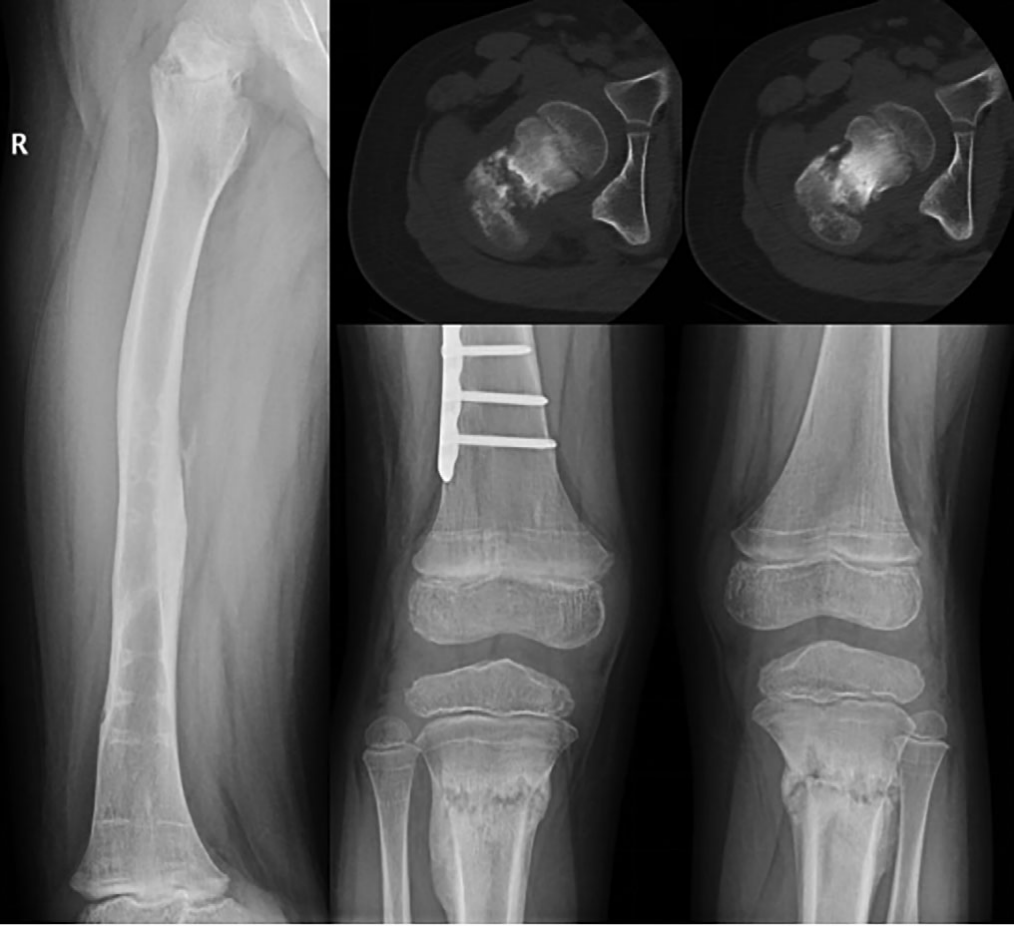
Twelve weeks after the right femoral neck fracture was found, the positive lateral position of the X‐ray showed the formation of the femoral neck of the femoral neck; the CT showed the cystic change of the femoral neck; 4 weeks after the two‐sided proximal fracture of the tibia, the X‐ray showed that the growth of the positive‐position bone was higher than before.
